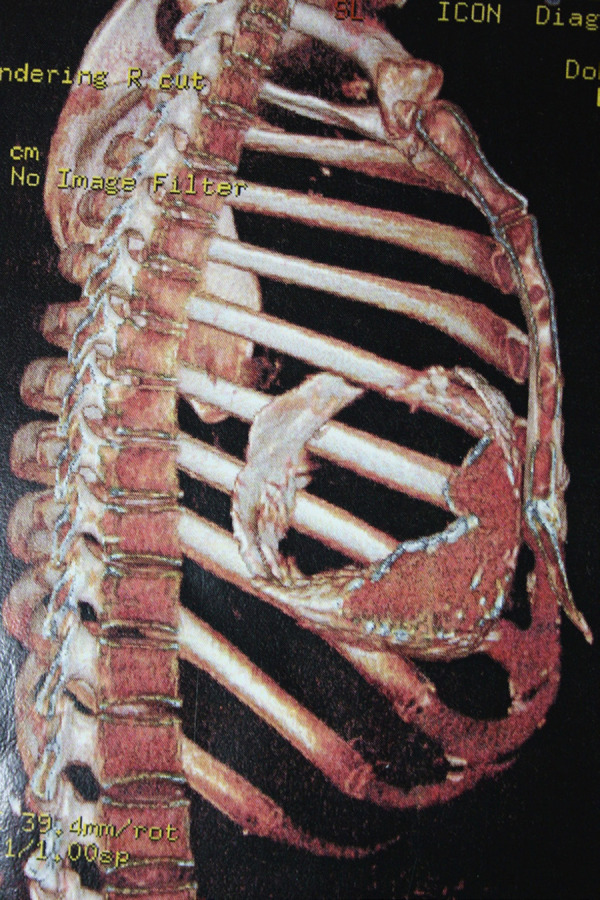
Magnetic resonance imaging of the chest.
